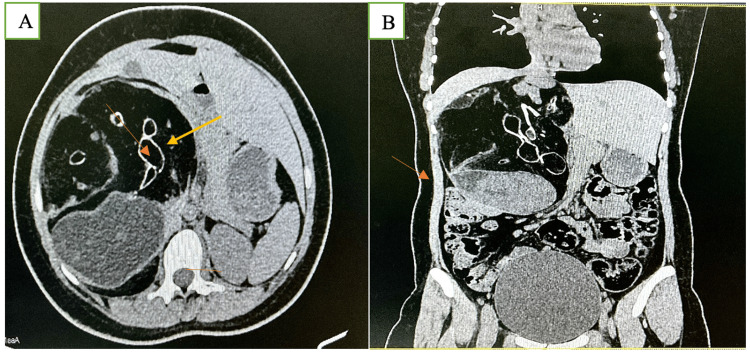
Axial CT images of a primary hepatic teratoma. In the provided CT images of a hepatic mass (presumably a mature teratoma), the arrows in image A mark specific characteristic components. The yellow arrow points toward the fat component of the lesion, seen as hypodense (dark) areas on non-contrast CT, consistent with mature adipose tissue within the teratoma. The orange arrowhead indicates calcification, appearing as a hyperdense (bright white) speck—typical of teeth or bone-like structures often found in teratomas. The brown arrow highlights a fluid or soft tissue density, potentially corresponding to sebaceous or keratinous material, or early solid elements. In image B (coronal post-contrast CT), the orange arrow likely points to an enhancing septation or mural nodule, representing the Rokitansky protuberance, a diagnostic feature comprising solid elements like hair follicles or glandular tissue. These areas enhance with contrast and are key in confirming the diagnosis of a complex teratoma.
